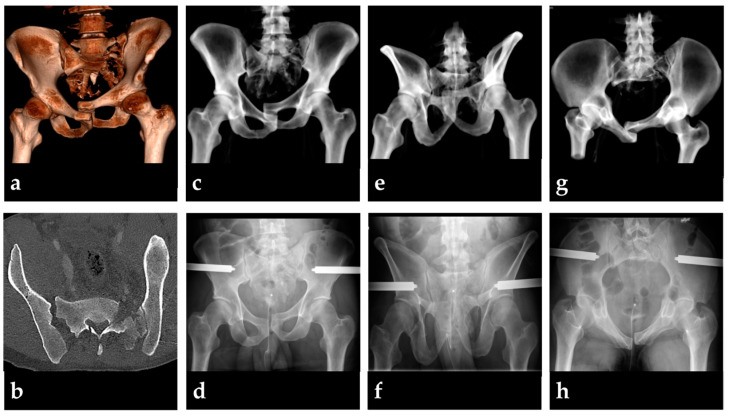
Example of an unstable pelvic fracture in a 40-year-old patient presenting at the emergency department with hemodynamic instability after falling from a 15 m height (ISS = 50, GCS = 13, initial lactate 5.8 mmol/L). A pelvic binder was placed in the prehospital phase and resulted in overreduction. A 3D reconstruction of the initial CT scans with the binder in place reveals the vertical shear pelvic injury pattern (a), with gross posterior instability resulting from bilateral transforaminal sacral fracture and unilateral sacroiliac dislocation (b). Initial fracture reduction and application were successfully performed using a pelvic C-clamp as shown in a.p., outlet, and inlet projections before (c,e,g) and after application (d,f,h). Application resulted in significant increases of sABP and MAP and a decrease in HR and SI, respectively (*p* < 0.05).
